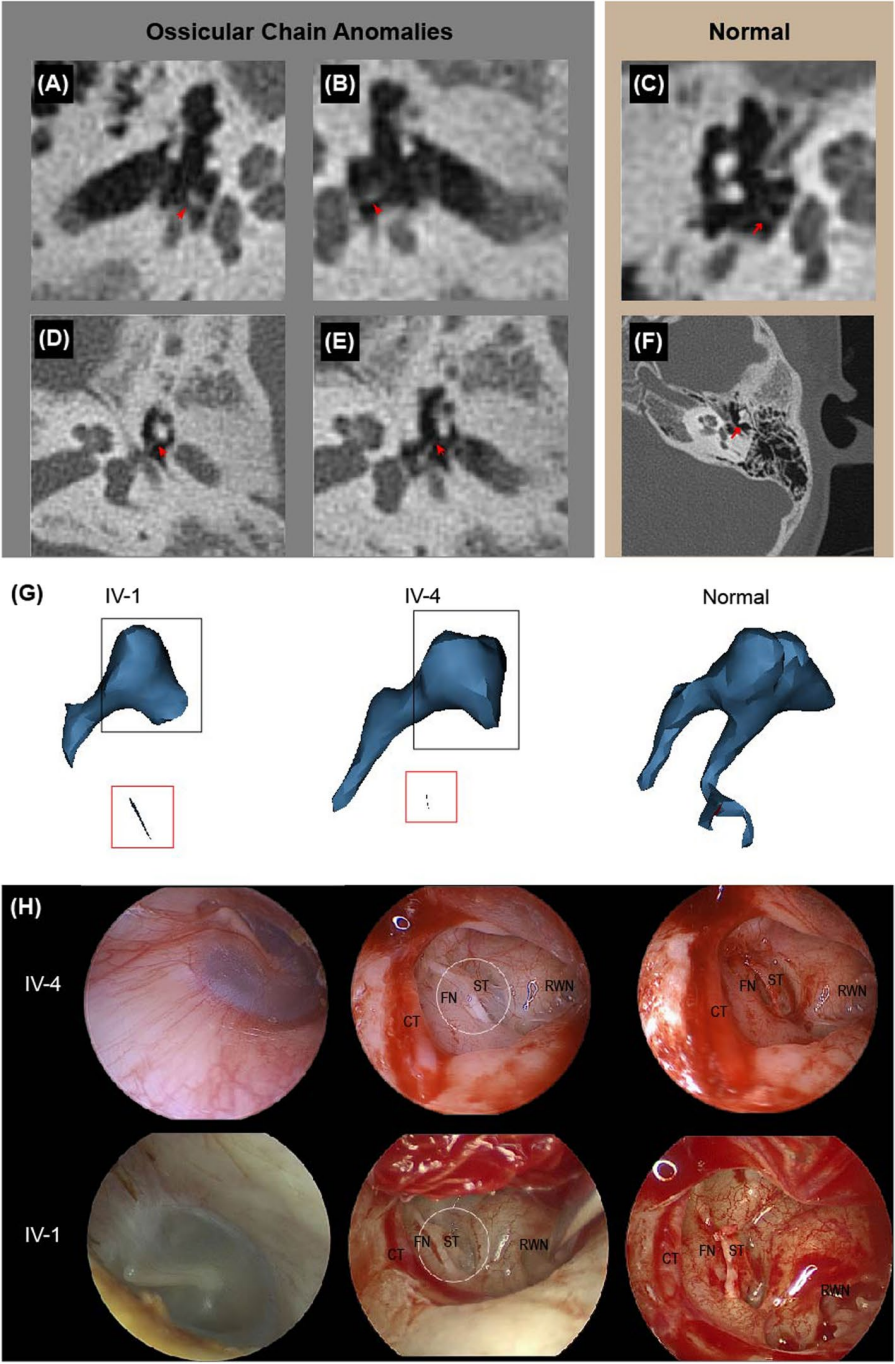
Ossicular chain malformations in IV-1 and IV-4. A-F CT scans of the TCS proband revealed ossicular chain anomalies. A, B Bilateral fusion of the anterior and posterior stapedial crura. C A normal stapes. D, E Malformation of the long crus of the incus. F A normal long crus of the incus. G Three-dimensional reconstruction of the ossicular chain of IV-1, IV-4 and normal person. Red block: stapes without footplate; Black block: malformation of incus H Intraoperatively, the ossicular chain anomalies in IV-1 and IV-4 were similar. CT: chorda tympani; FN: facial nerve; ST: stapes; RWN: round window niche
